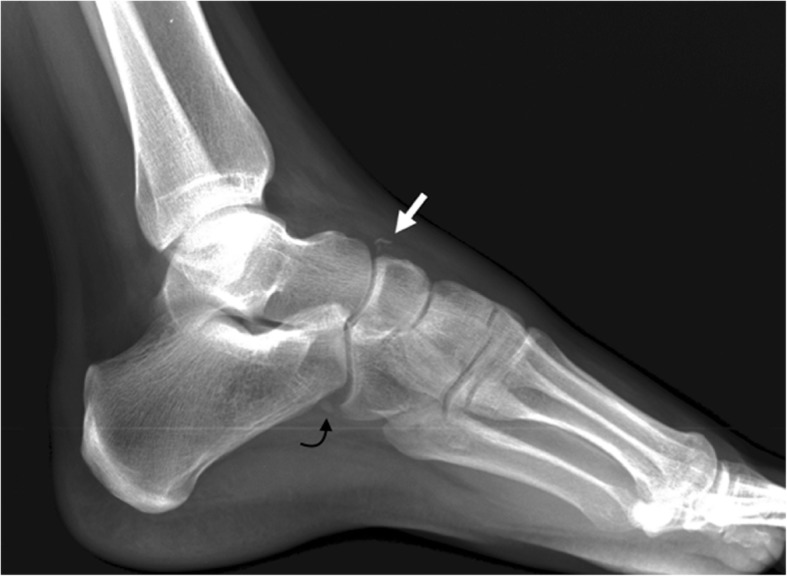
Differential diagnosis of os supranaviculare. The differential diagnosis has to be established with an avulsion fracture of the talo navicular joint capsule, as seen in this lateral radiograph (white arrow). Note the mild swelling of the soft tissues associated and the more linear configuration of the fragment, compared to the more triangular shape of the ossicle. Incidental note os peroneum (curved black arrow)
